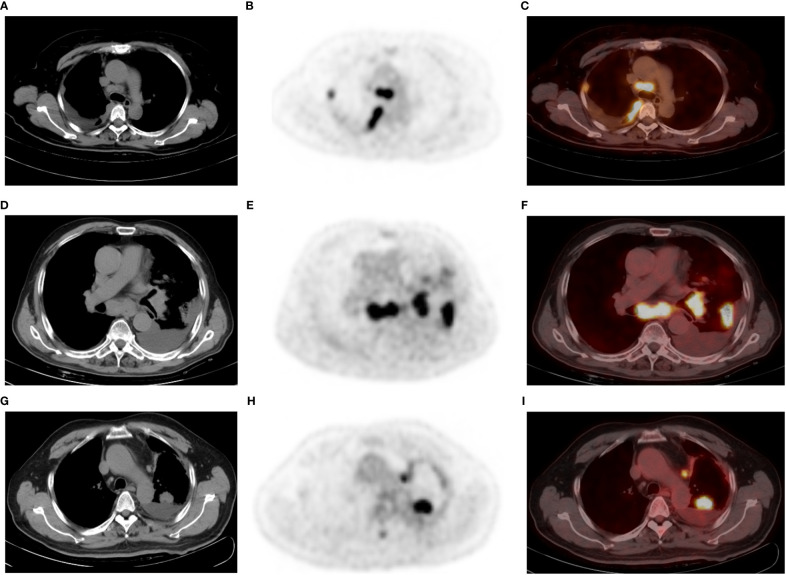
A 52-year-old man with poorly differentiated lung adenocarcinoma and right-sided malignant pleural effusion (A–C). Axial CT (A) revealing effusion in the right pleural cavity, and axial ^18^F-FDG PET (B) and fused PET/CT (C) revealing nodular ^18^F-FDG uptake (SUVmax 4.4) in the same pleural region. Thoracentesis-based pathology confirmed MPE caused by metastatic adenocarcinoma. A 77-year-old man with lung adenocarcinoma and left-sided BPE (D–F). Pleural fluid cytologic findings were negative, without recurrence of pleural abnormalities on follow-up. Axial CT (D) revealing a left peripheral lung cancer with left pleural effusion, as well as pneumonia, mediastinal, and left hilar lymphadenopathy. Axial PET (E) and fused PET/CT (F) revealing hypermetabolic areas in the tumors without abnormal FDG uptake in the pleura. A 65-year-old man with left lung adenocarcinoma and left-sided MPE (G–I), who did not have an enlarged lymph node in the mediastinum or hilar region. Axial CT (G), PET (H) and fused PET/CT (I) revealing a left PE, lung mass (SUVmax: 13.9), and mediastinal pleural thickening (thickness: 5.6 mm and SUVmax: 4.1). PE cytology confirmed MPE. Note that the largest thoracic lymph node of this patient is located next to the aortic arch with a short diameter of 7 mm and SUVmax 6.5.
